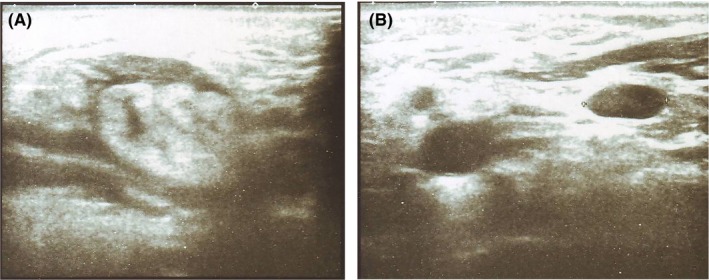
Ultrasound features of the (A) affected submandibular salivary gland and (B) lymph nodes
